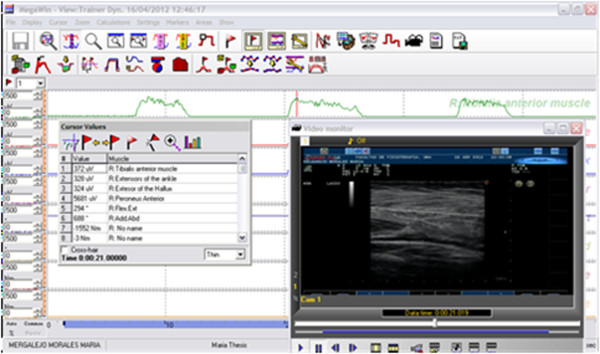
Synchronization example of ultrasound images and sEMG muscle activity.
